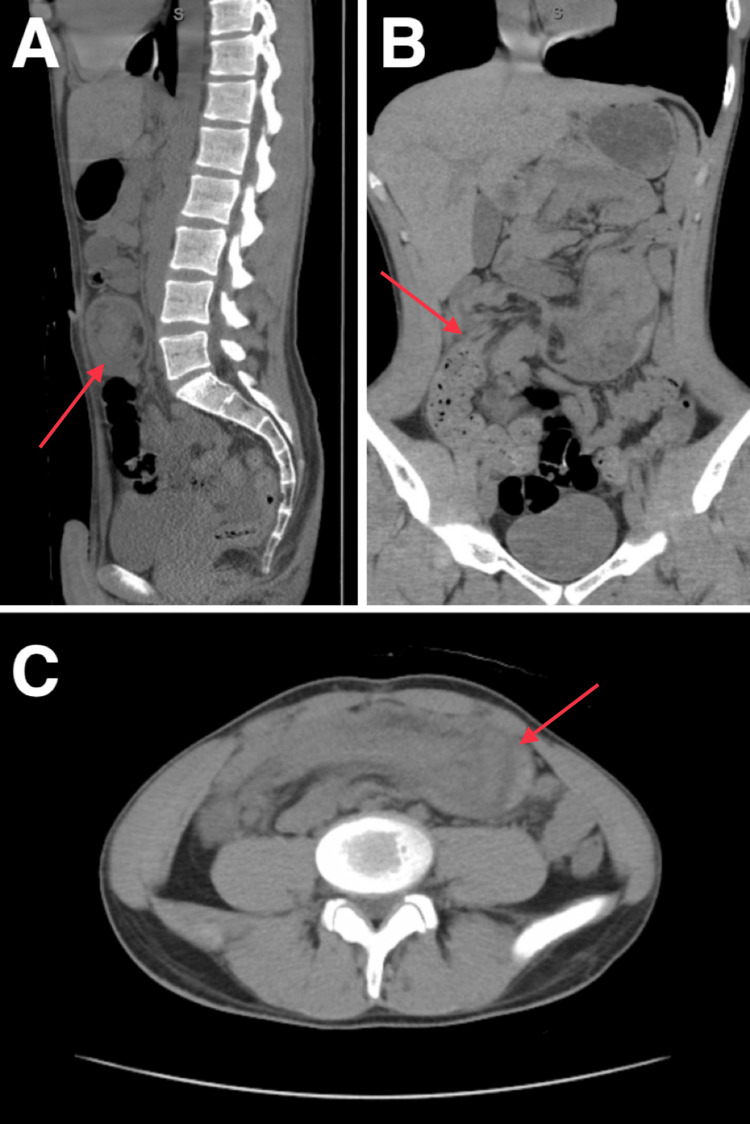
Non-contrast computed tomography of the abdomen and pelvis (A) Coronal view demonstrating intussusception of the ileocecum into the transverse colon with resultant small bowel obstruction. (B) Sagittal view correlating the intussusception finding. (C) Axial view correlating the intussusception finding.
